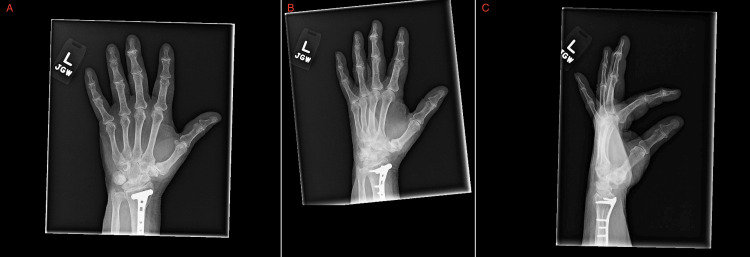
Multiview Radiographs of the Left Hand Demonstrating Chronic Degenerative Changes Anteroposterior (A), oblique (B), and lateral (C) radiographic views of the left hand demonstrate findings consistent with chronic degenerative joint disease. There is a partially visualized distal radial fixation plate with screws, consistent with prior surgical intervention. Mild joint space narrowing is seen at the radiocarpal joint. Notably, there is moderately severe joint space narrowing with osteoarthritic changes at the first carpometacarpal (CMC) joint, consistent with thumb base osteoarthritis. Additional degenerative changes are observed at multiple distal interphalangeal (DIP) joints, most pronounced in the third digit. No acute fracture, dislocation, or soft tissue abnormality is identified.
